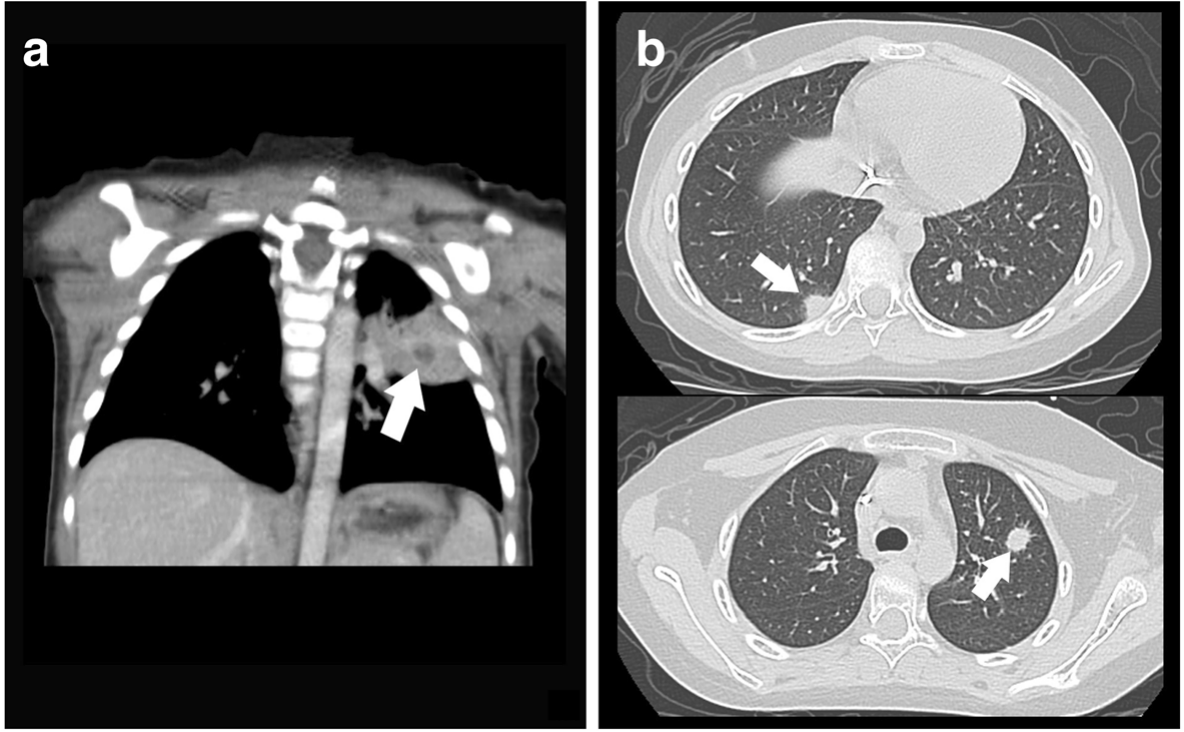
Computed tomography of the lungs. a In Patient 1, consolidation in the left lower lobe was noted (*white arrow*). b In Patient 2, there were nodules in the right middle and left upper lobes (*white arrows*)
